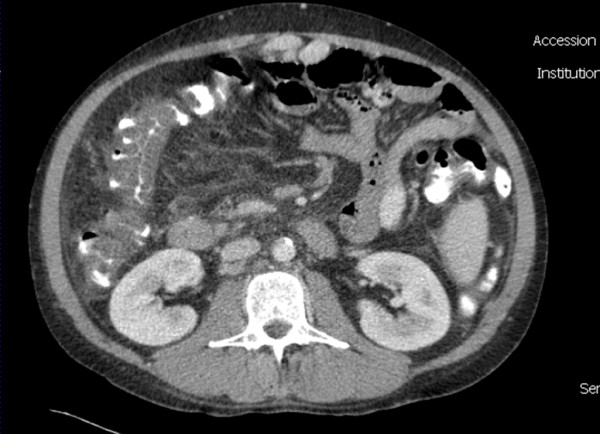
CT scan of case 1 showing extensive oedema of the right a transverse colon, as well as cirrhotic alterations of the liver.
